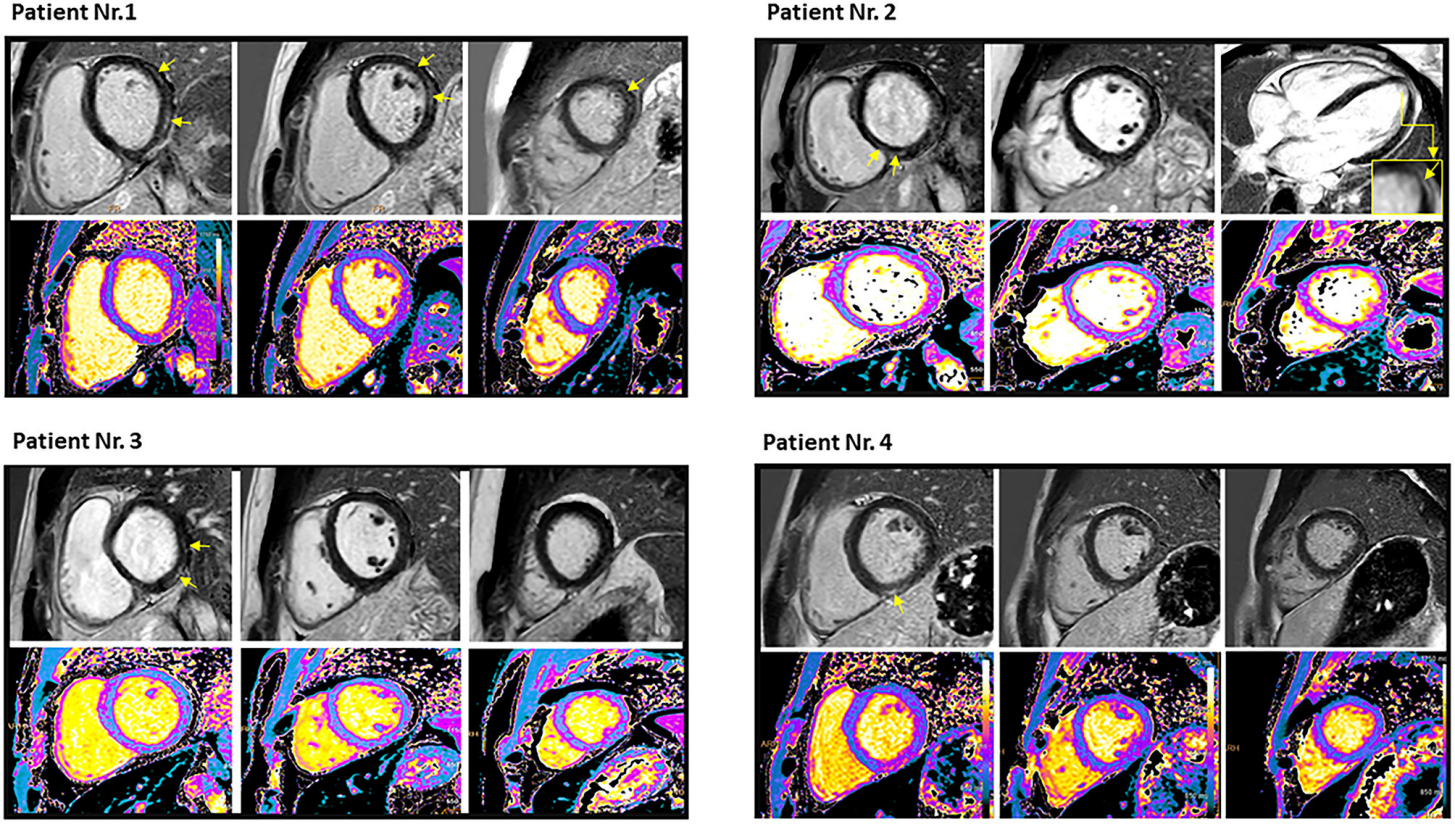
Cardiac magnetic resonance imaging of the four patients who had myocarditis following the third dose of mRNA COVID-19 vaccination demonstrated late gadolinium enhancement (yellow arrows) and T1 mapping (lower row). Patient no. 1: Mid wall late gadolinium enhancement involving 9% of the myocardium with corresponding myocardial injury in native T1 mapping imaging in antero- and infero-lateral segments of basal and mid ventricular short-axis view, as well as in the lateral segment of apical short-axis view. Native T1 value was 1,135 ms, and T2 value was 69.2 ms. Peak troponin was 240 ng/L, and scan delay (from COVID-19 vaccine) was 8 days. Patient no. 2: Mid wall late gadolinium enhancement involving 1% of the myocardium with corresponding myocardial injury in native T1 mapping imaging in the lateral segment of apical and in the septal segment of the basal short-axis view. Native T1 value was 1,077 ms, and T2 value was 57.5 ms. Peak troponin was 80 ng/L, and scan delay (from COVID-19 vaccine) was 40 days. Patient no. 3: Epicardial late gadolinium enhancement involving 3% of the myocardium with corresponding myocardial injury in native T1 mapping imaging in the inferior and inferolateral segments of the basal short-axis view. Native T1 value was 1,155 ms, and T2 value was 58.1 ms. Peak troponin T was 4,967 ng/L, and scan delay (from COVID-19 vaccine) was 47 days. Patient no. 4: Mid wall late gadolinium enhancement involving 1% of the myocardium with corresponding myocardial injury in native T1 mapping imaging in inferior segments of the basal and mid-ventricular short axis view, as well as in the lateral segment of the apical short axis view. Native T1 value was 1,041 ms, and T2 value was 57.4 ms. Peak troponin T was79 ng/L, and scan delay (from COVID-19 vaccine) was 42 days. Reference (normal) values: T1: 950–1,060 ms, T2: < 57 ms, and troponin T < 13 ng/L.
